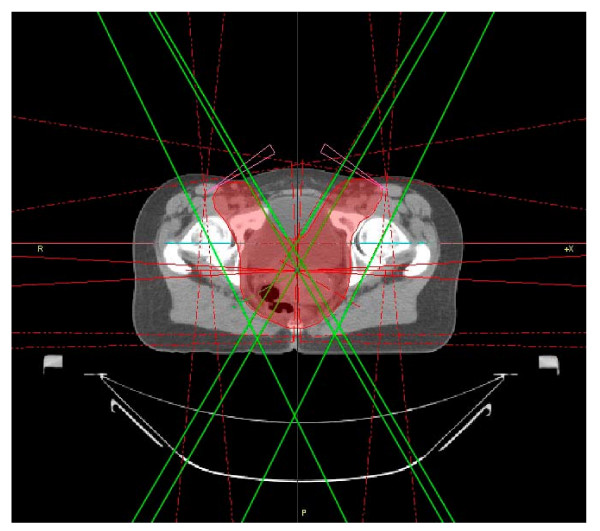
Axial CT for 3D-CRT with PTV and 6 beams.
